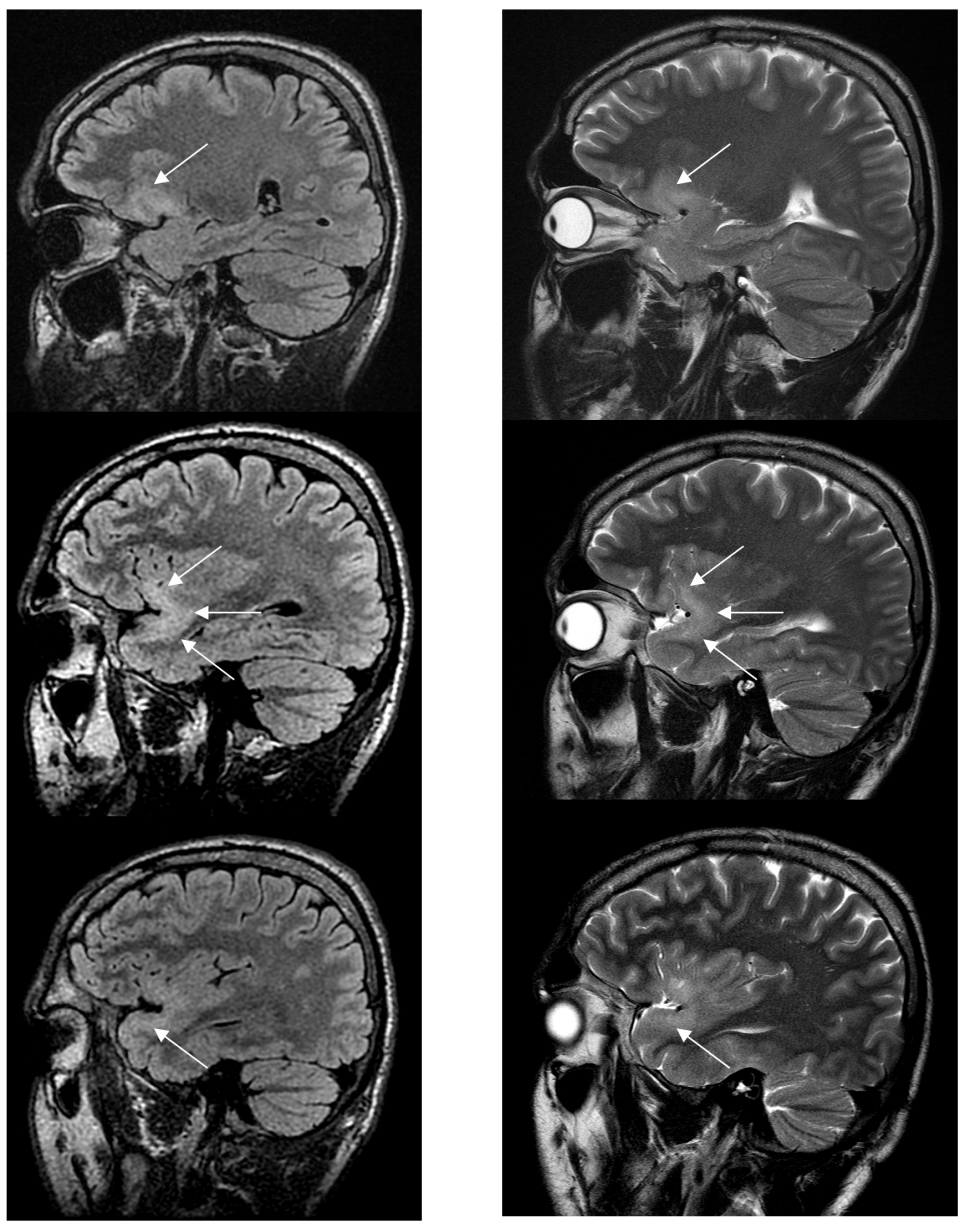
Sagittal FLAIR-weighted MRI (left) and sagittal T2-weighted MRI (right) performed in a 30-year-old right-handed asymptomatic patient who participated in a research protocol. This MRI shows a left WHO grade II glioma involving the uncinate fascicle (white arrows), from the orbitofrontal part (upper) to the temporopolar part (lower) through the temporal stem (middle).
